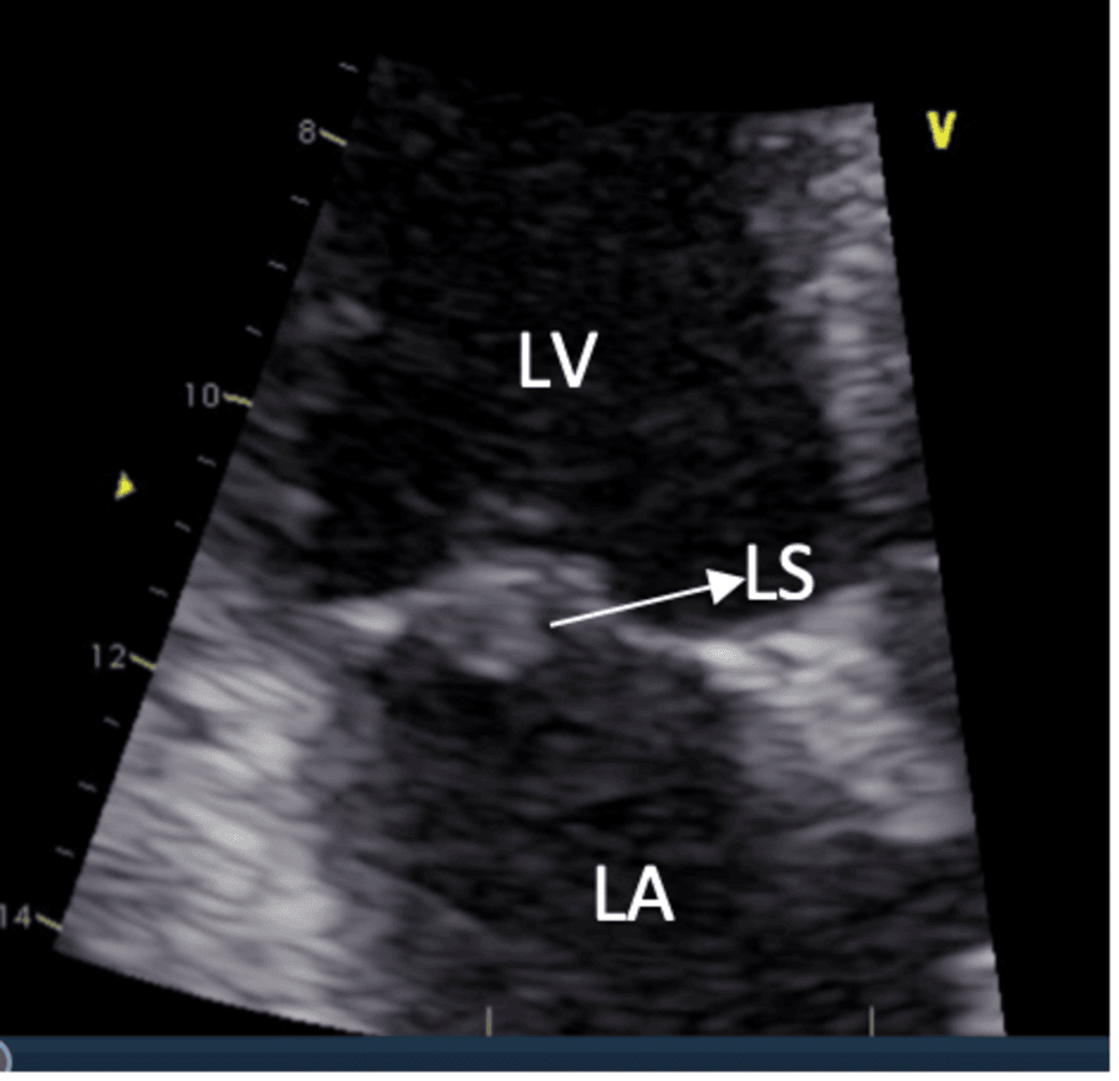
TTE showing an oscillating mass on the posterior leaflet of the mitral valve LA, left atrium; LV, left ventricle; LS, Libman-Sacks endocarditis; TTE, transthoracic echocardiogram
